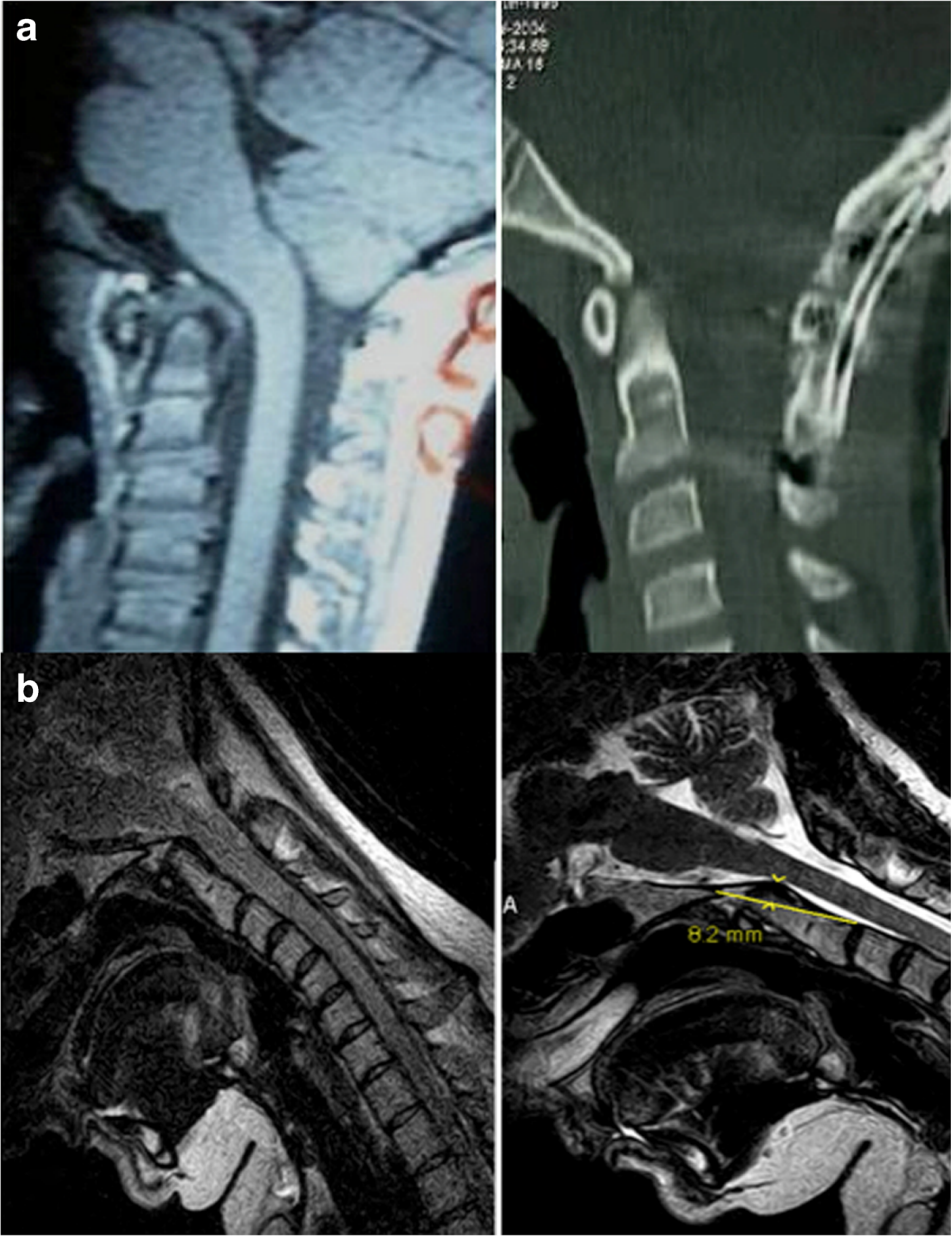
a Preoperative and postoperative CT of a patient showing correction of the CXA and stabilization of the craniocervical junction. b Preoperative and postoperative MRI in the flexed position showing the results of intraoperative correction of the CXA to straighten the neuraxis and thereby reduce the neuraxial strain
